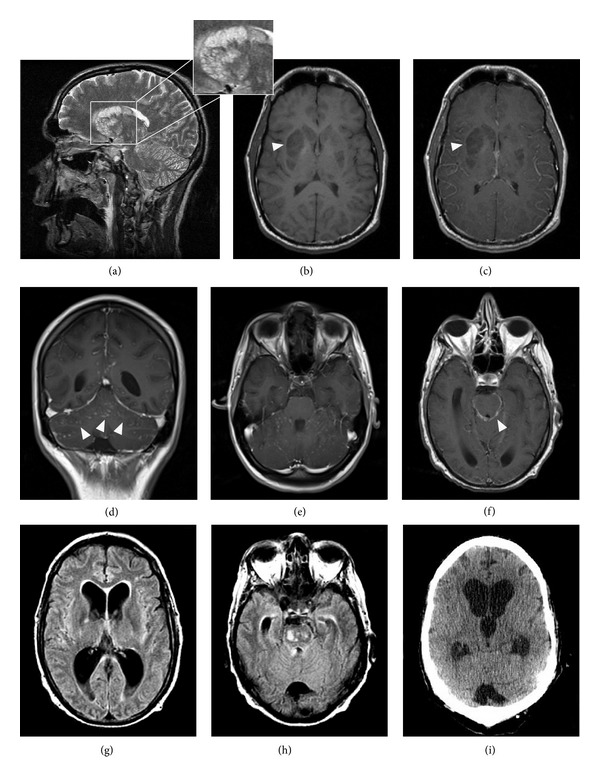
Examples of abnormal neuroradiological findings in cryptococcal meningoencephalitis. (a) presents the typical pattern of cryptococcal gelatinous pseudocysts located in lentiform and caudate nuclei (T2WI hyperintense signal) while T1WI sequences ((b), arrow head) show a hypointense signal without enhancement in the T1 C+ image ((c), arrow head). Signs of meningitis are shown by leptomeningeal enhancement in the cerebellar Gyri in axial ((d), arrow heads) and coronal (e) T1 C+ scans. (f) presents signs of basal meningitis demonstrated by leptomeningeal enhancement (arrow head). Hydrocephalus is shown in axial FLAIR sequences in (g) and (h). The enlargement of the frontal and dorsal horns of the lateral ventricles with a hyperintense rim of the periventricular white matter indicates a CSF extravasation. A CCT scan performed 5 days later shows increased ballooning of the third ventricle and a progressive enlargement of the lateral ventricles (i).
